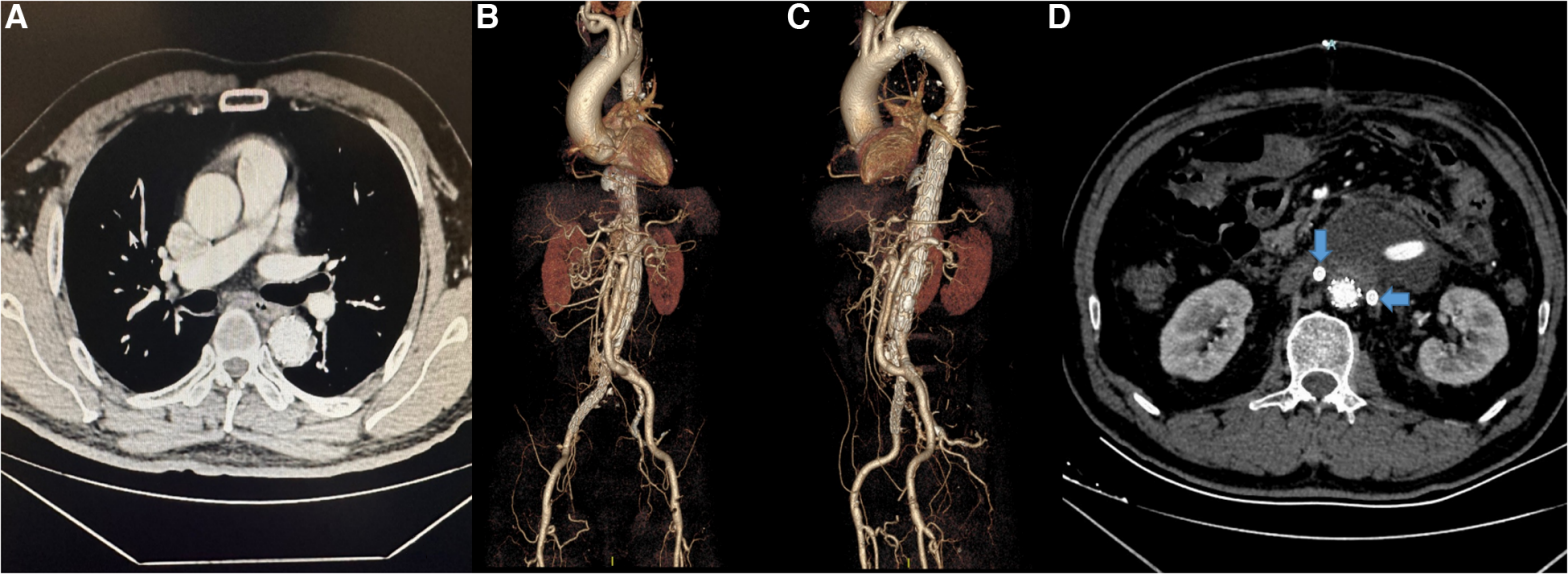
(A–C) The CTA and 3-dimensional reconstruction of patient after surgery. (D) The bilateral renal arteries (arrows) were patent.
